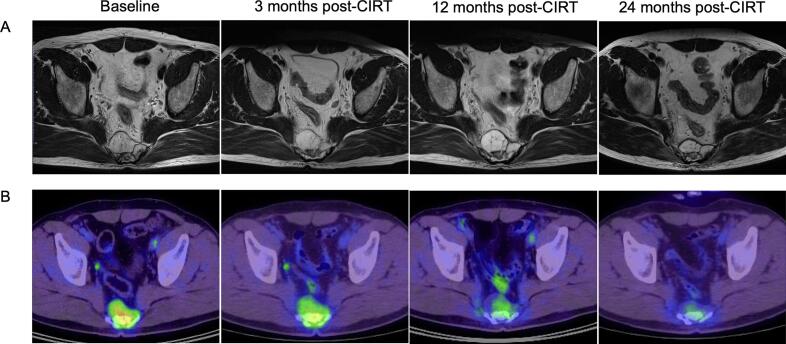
Representative case of tumor response in a patient with local control. MRI and FDG-PET/CT images of a 55-year-old male with sacral chordoma treated with CIRT. A: MRI-T2WI, axial plane. The LAD of the tumor decreased by 0%, 0% and 6% at 3, 12 and 24 months post-CIRT, respectively. B: FDG-PET/CT-axial plane. SUV_max_ decreased by 25%, 45% and 62% at 3, 12 and 24 months post-CIRT, respectively.
